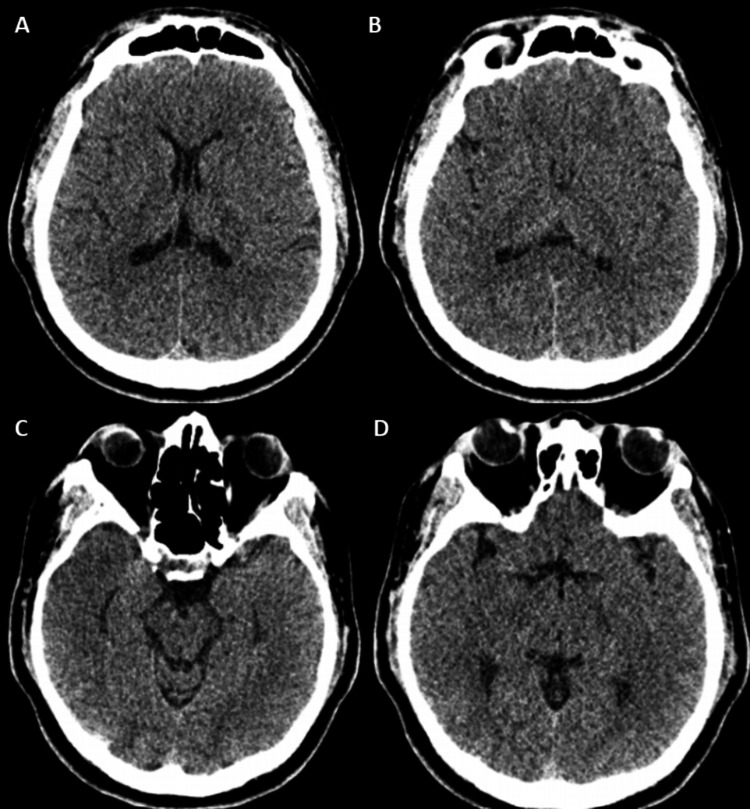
Plain CT of the brain did not reveal any abnormalities. (A-D) No significant abnormalities were detected. CT: computed tomography
